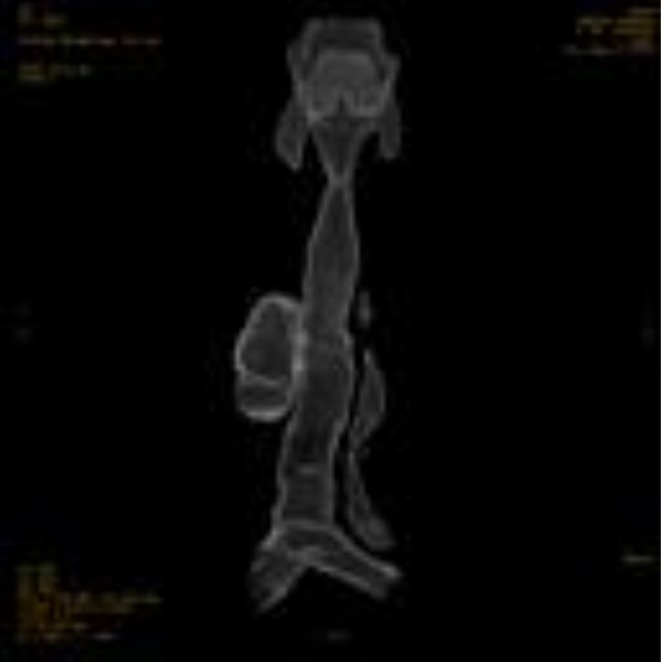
3D CT reconstruction clearly visualizes the tracheal diverticulum and its anatomical relationship to the trachea. This reconstruction was invaluable for surgical planning.
